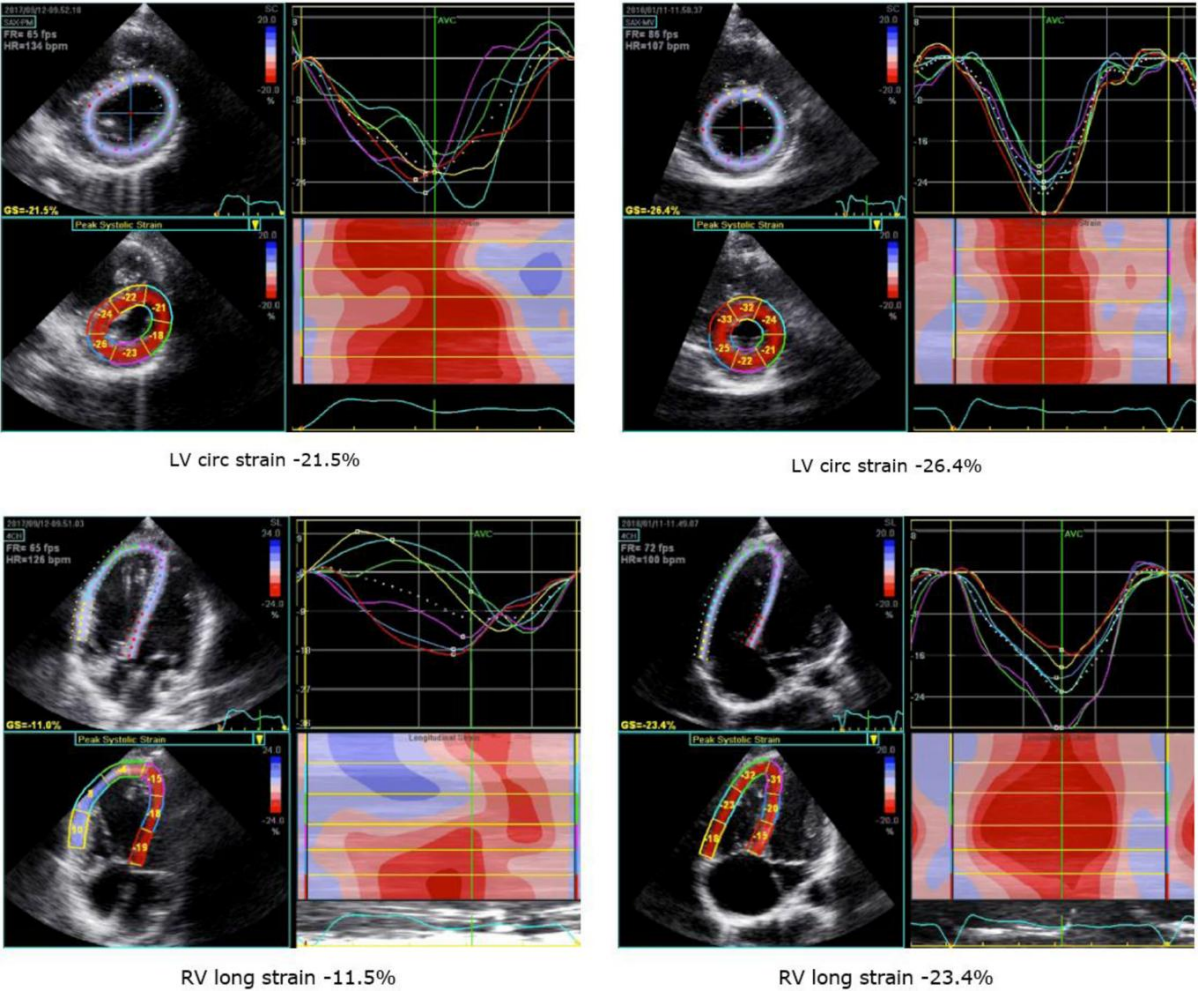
Exercise stress echocardiogram at 100 W, left footballer, and right tennis player.
